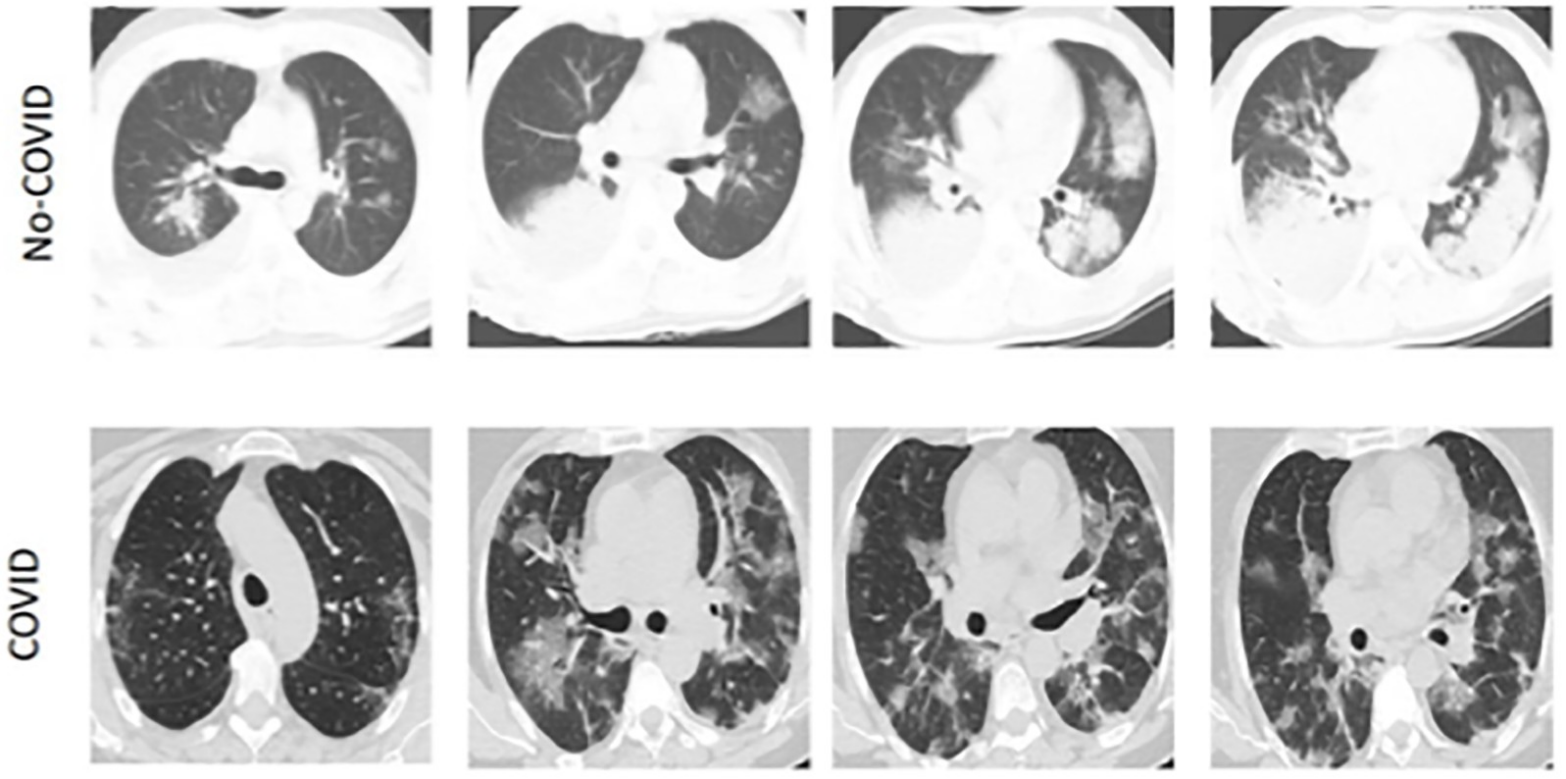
The appearance and contrast of CT images from COVID-19 patients vary significantly across two different clinical centers, indicating data heterogeneity.
